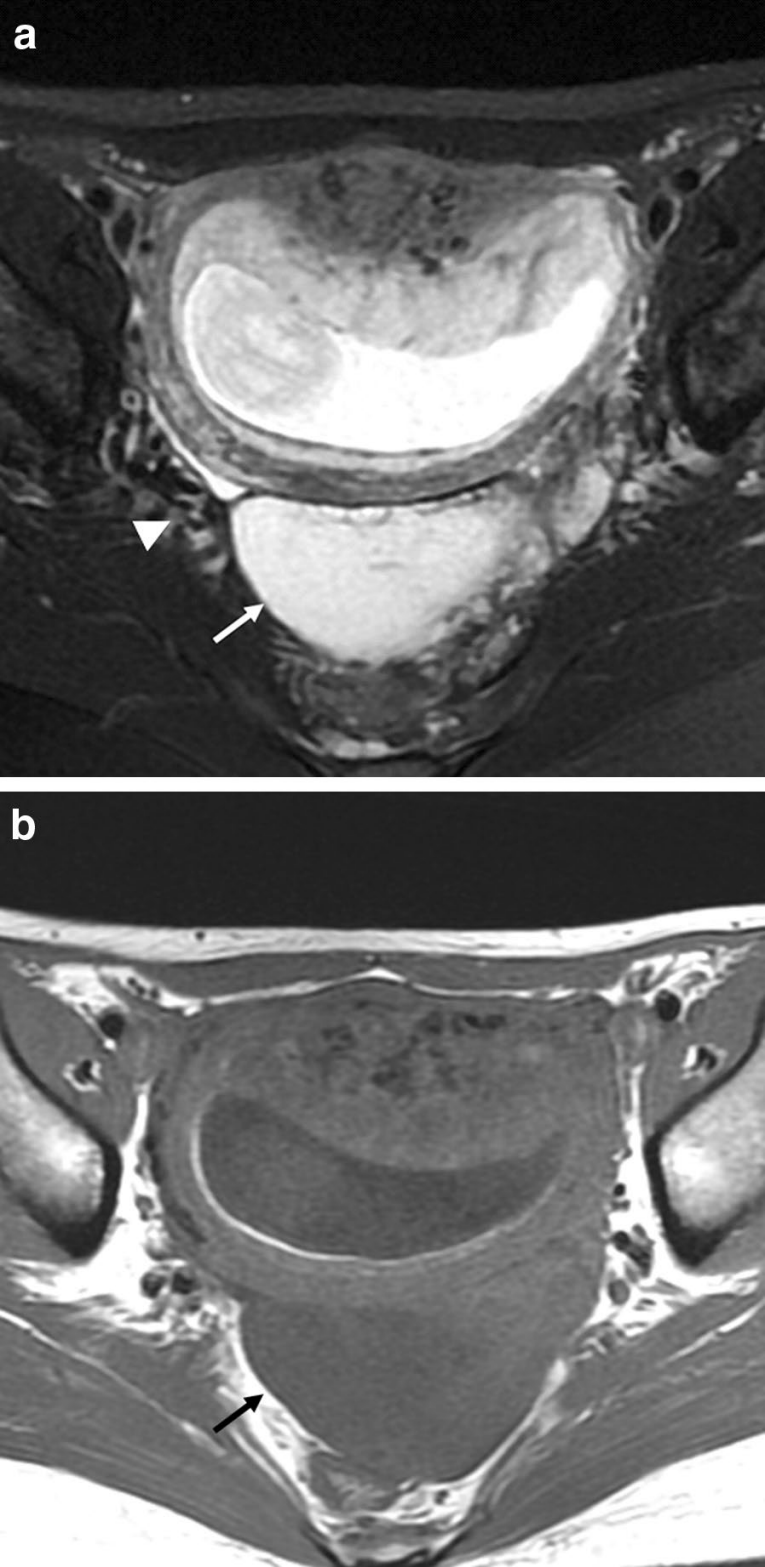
a Fat-suppressed T2-weighted image shows the markedly high intensity mass (*arrow*) with peripherally displaced follicles. The ovary has a teardrop configuration and the vascular pedicle appears twisted (*arrowhead*) beside the enlarged ovary and uterus. b T1-weighted image shows a homogenous low-intensity mass (*arrowhead*)
